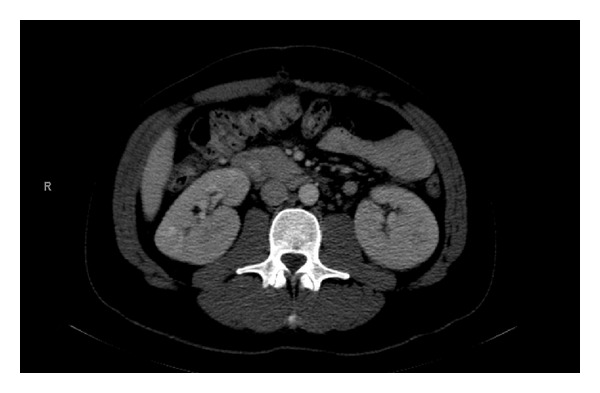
CT scan with oral contrast showing enlarged 15 cm right kidney and 14.5 cm left kidney with no hydronephrosis.
